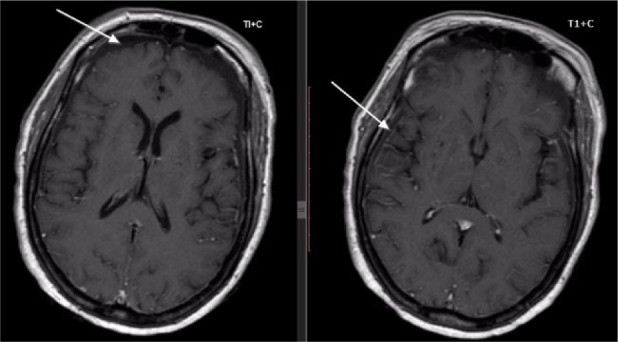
Brain MRI (T1+ C) showing bilaterally thin but widely spread subdural collection with peripheral meningeal enhancement in the frontoparietal region. Leptomeningeal enhancement is also seen.
